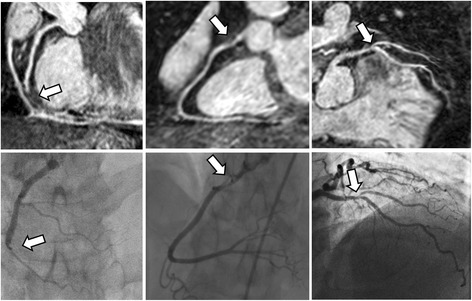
Images from three patients with coronary artery disease, diagnosed using coronary magnetic resonance angiography (top row) and confirmed with coronary x-ray angiography (bottom row)
